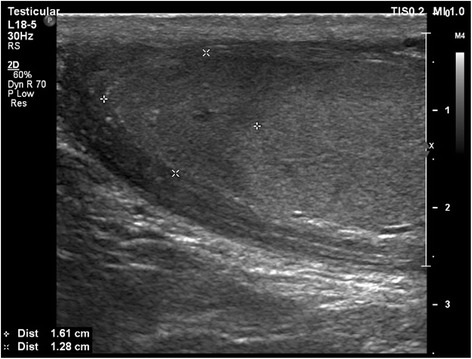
Ultrasonography showing a remaining hypofixation of the upper pole at follow-up
